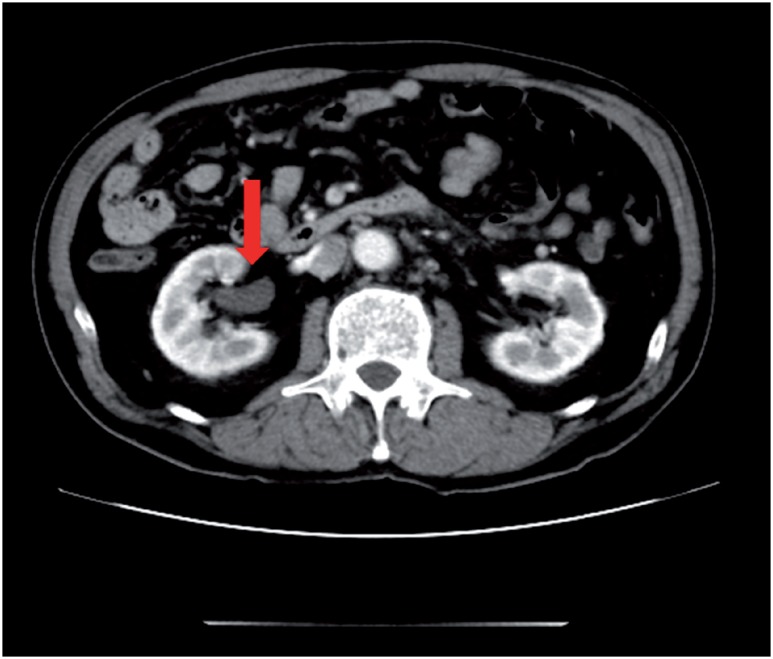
B) Enhanced computed tomography also showed a slight right hydronephrosis.
